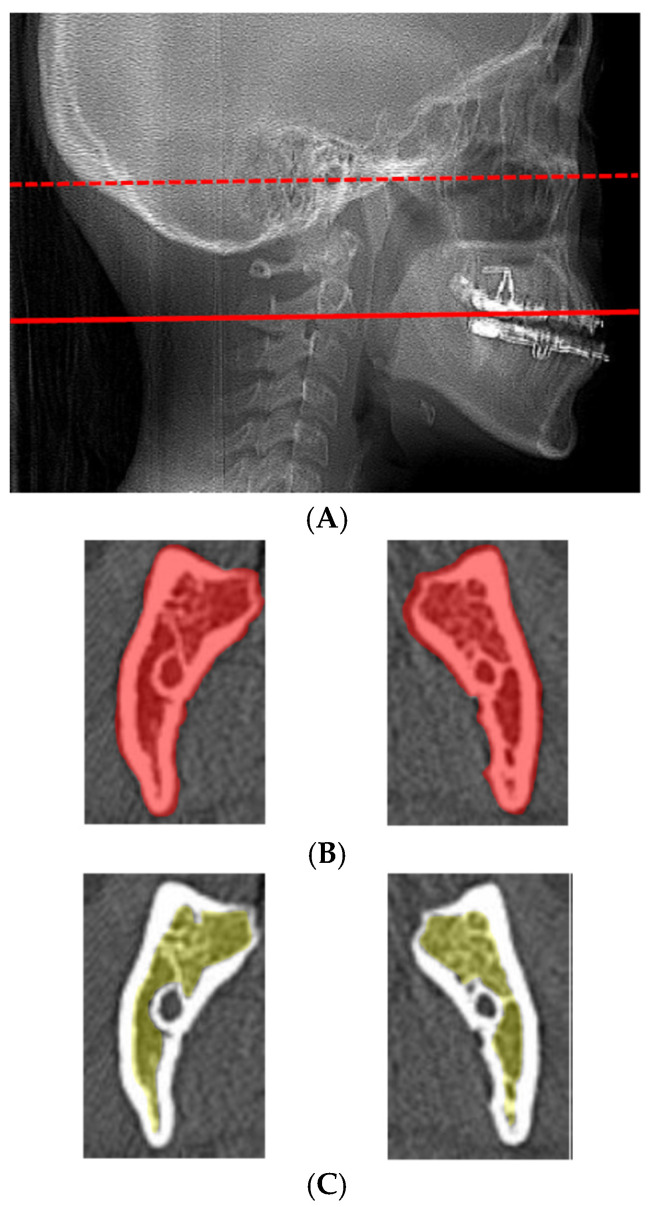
Mandibular ramus measurements. (A) Lateral CT for measurements of the mandibular body. FH plane, dotted line; measurement position, solid line. (B) Cortical and cancellous bone is extracted from axial-view CT of the mandibular ramus (red). (C) Cancellous bone is extracted from axial-view CT of the mandibular body (yellow). ROIs were placed in the mandibular ramus, including cortical and cancellous bone, avoiding soft tissues and teeth. A slice parallel to the FH plane through the second molar was used, and left and right sides were averaged.
